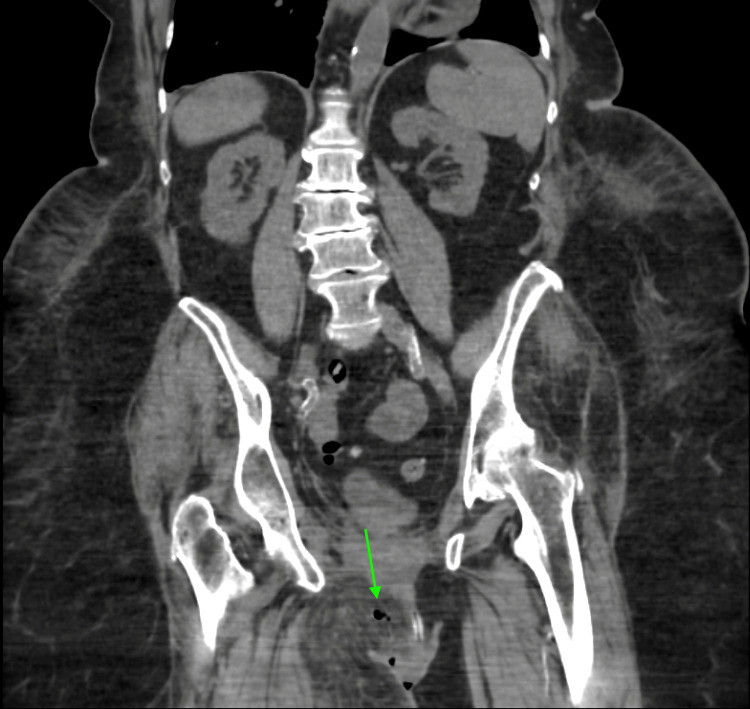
CT scan (coronal cut) showing right-sided gas in the connective tissue.
